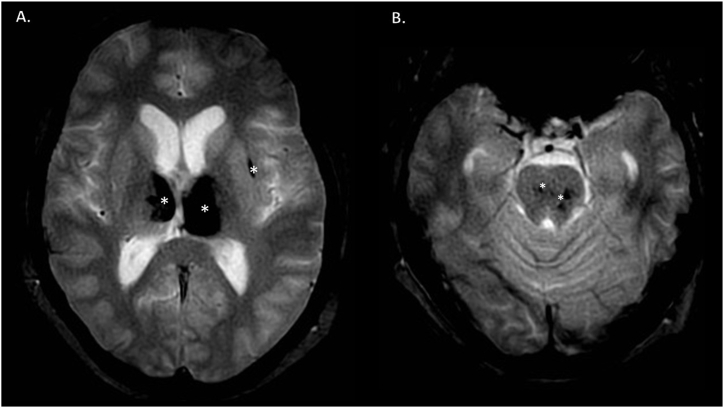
MRI T2* Fast field echo (FFE) of bilateral thalamic and pons bleeding. A: bilateral thalamic and external capsule bleeding (white stars). B: small hemorrhages in pons (white stars).
